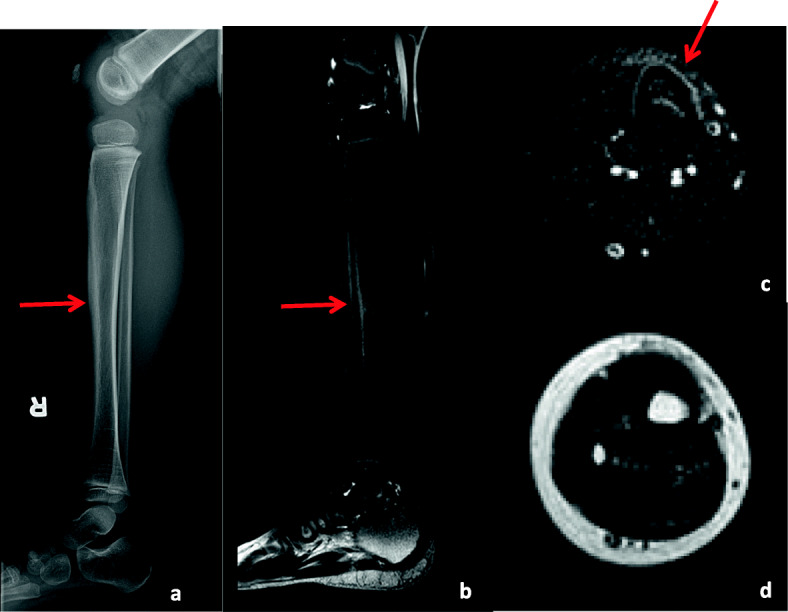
Case 4 Lateral radiograph of the right lower extremity (a) shows linear subperiosteal new bone formation along the mid tibial diaphysis The fat-saturated sagittal (b) and axial (c) T2 MR images of the right lower extremity obtained 4 months after the aforementioned x-rays show periosteal reaction along the mid right tibial diaphysis associated with increased signal intensity within the underlying cortex and bone marrow and at a lesser extent, anterior soft tissues (arrows). Low signal intensity is noted in the corresponding regions of the axial T1 (d) MR image. No discrete fracture line is noted
